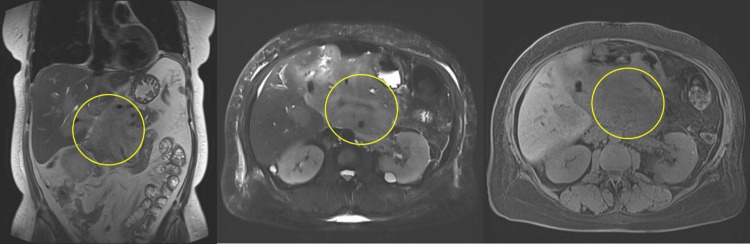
Coronal (left), axial (center), and axial (right) magnetic resonance images (MRCP protocol, no IV contrast) of the abdomen Coronal (left), axial (center), and axial (right) MR images (MRCP protocol, no IV contrast) demonstrate a large lesion (circled) arising from the porta hepatis and encompassing the pancreatic head. The mass extends along the posterior wall of the stomach and involves the first and second portions of the duodenum. Note the dilated pancreatic duct, which remains visible in its entirety, despite the extensive involvement by the lesion.​ MRCP, Magnetic Resonance Cholangiopancreatography
